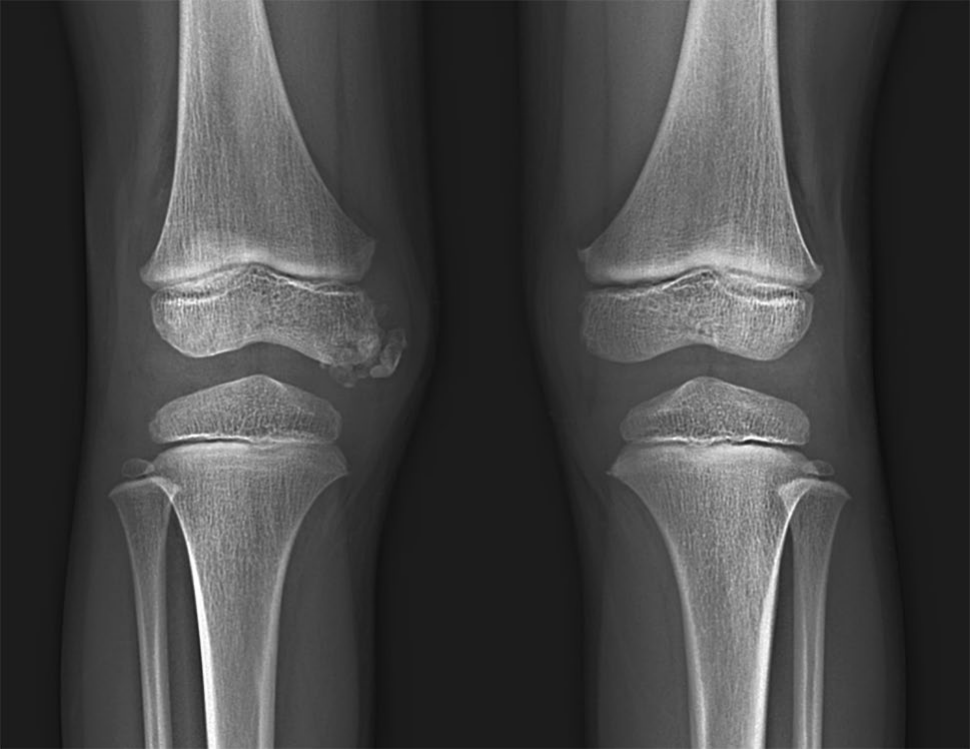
A 5-year-old boy with dysplasia epiphysealis hemimelica (Trevor’s disease). Radiograph shows osseous overgrowth arising from the right medial femoral condyle
